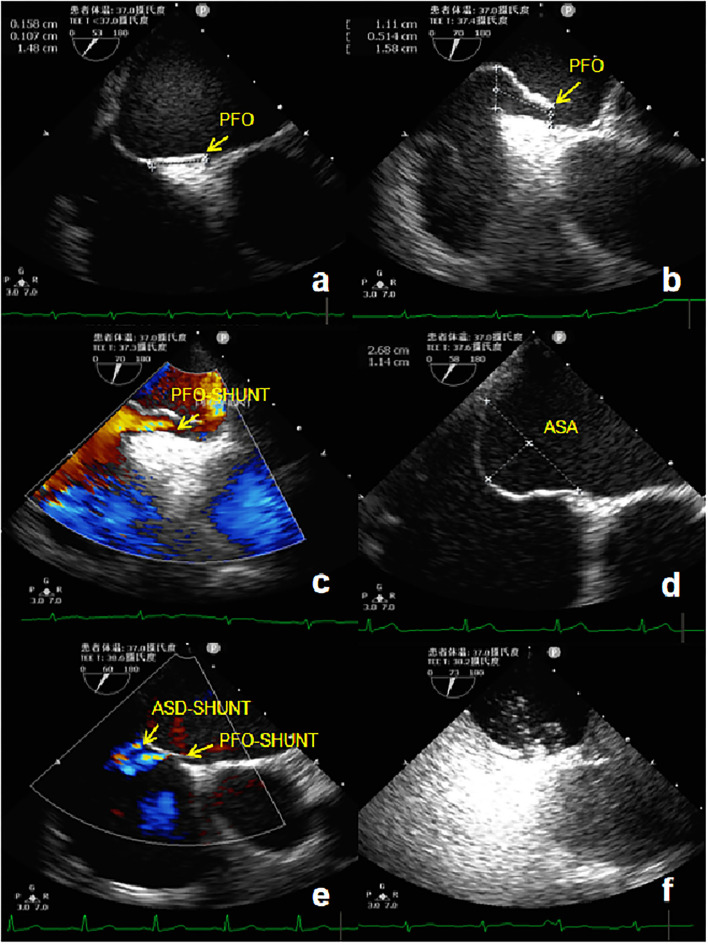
TEE detection of PFO. (a) Two-dimensional TEE displaying a “slit-like” channel of PFO at rest. (b) Two-dimensional TEE displaying a “slit-like” channel of PFO during Valsalva maneuver. (c) Doppler color flow imaging showing spontaneous PFO left-to-right shunt. (d) Two-dimensional TEE displaying an ASA. (e) Doppler color flow imaging showing spontaneous the left-to-right shunt of PFO and small ASD. (f) TEE bubble study showing microbubbles passing through the PFO. TEE, Transesophageal echocardiography; PFO, Patent foramen ovale; ASA, Atrial septal aneurysm; ASD, Atrial septal defect.
